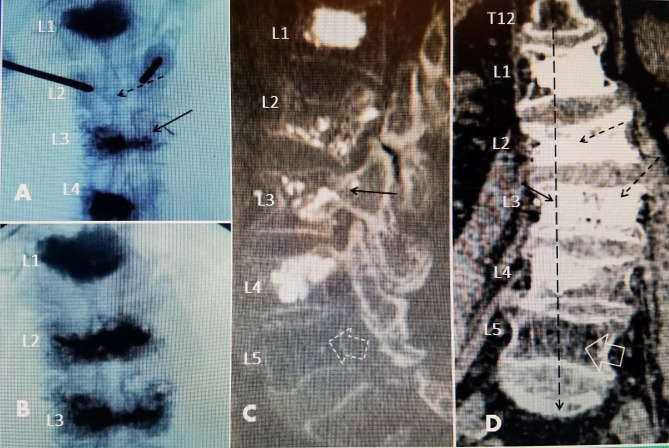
Reconstructed computerized tomography (CT) after four vertebroplasties with scoliosis A: Intra-operative fluoroscopy for L2 vertebral augmentation. The patient had redevelopment of pain with marked uptake at L2 on a bone scan (dashed black arrow) and smaller uptake L3 (solid black arrow), where insufficient cement from a previous vertebral augmentation had not completely filled the fracture. B: Postoperative film showing the filling of L2 and the additional filling of superior and lateral L3. C: Computed tomography (CT) after vertebroplasty at L3 (solid black arrow). Sagittal computerized tomography with bone window demonstrates the marked hypodensity of L5 (open, dashed, large white arrow), D: Coronal CT with soft tissue window showing complete filling of L2 and L3 ( dashed black arrows) with cement in the coronal plane. There is marked loss of bone trabecular pattern and hypodensity throughout the vertebral body of L5 (open, solid, large white arrow).The weight-bearing center of gravity relative to the lumbar scoliosis is indicated (long, dashed black arrow) passing from mid L1 to the lateral concave side of L2, L3, and L4 through the center of L5.
